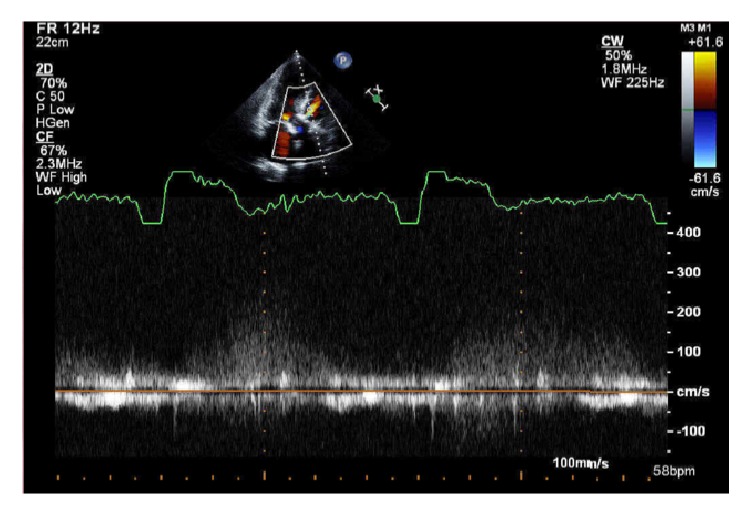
1-Year follow-up: TTE 2D ECHO with color flow shows the residual aorto-RV shunt with a continuous wave Doppler, which demonstrates significant reduction in the velocity compared to the initial measurements.
